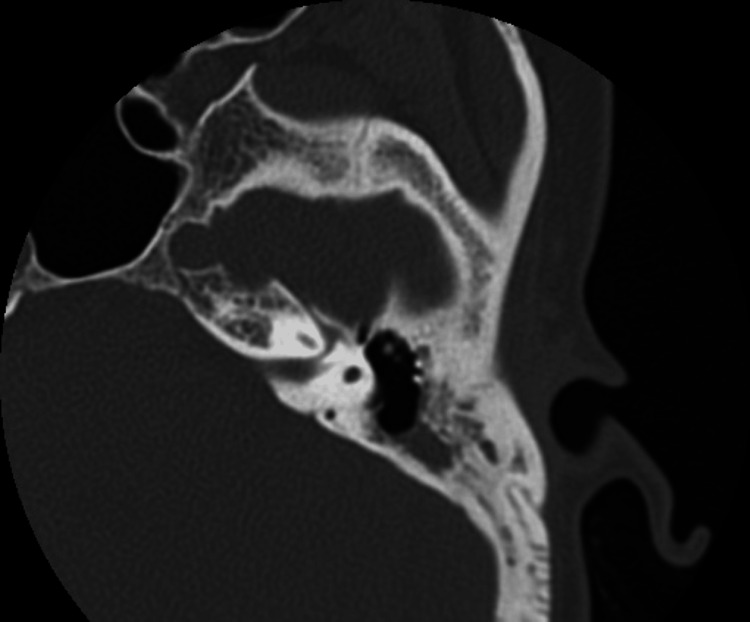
Sample error image of a misclassified SCOM CT scan image using the Logistic Regression model SCOM: suppurative chronic otitis media; CT: computed tomography.
